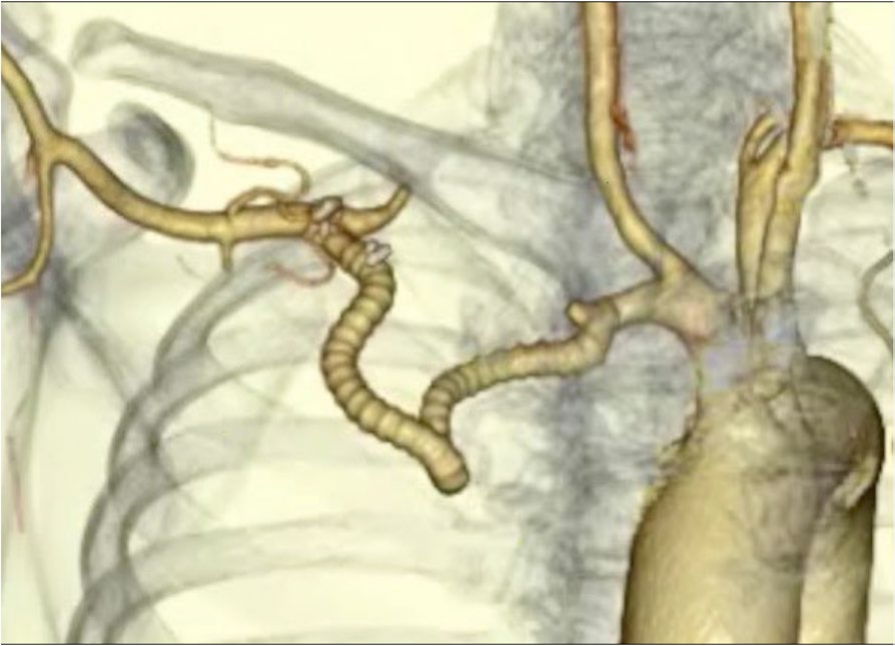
The 3D enhanced computed tomography image of the reconstruction of the right subclavian artery is shown
